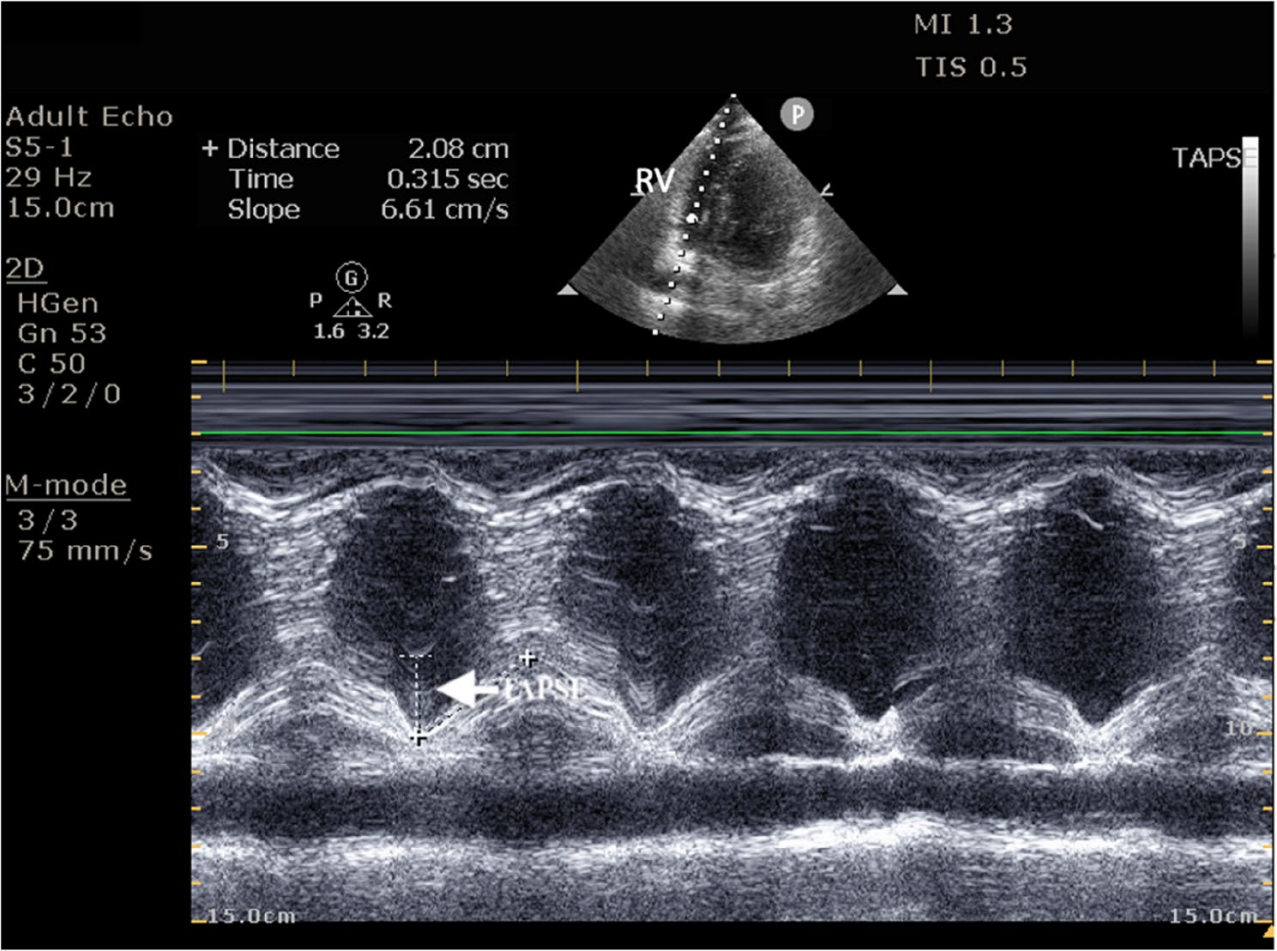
Echocardiographic measurement of TAPSE. Shows the measurement TAPSE (white arrow) depicted to be 20.8 mm. TAPSE = Tricuspid Annular Plane Systolic Excursion, RV = Right Ventricle
